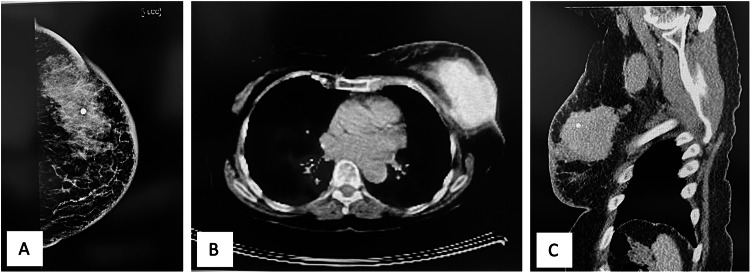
Left breast locally advanced TNBC. A: Left breast mammogram. B: PET-CT showing extensive FDG uptake in the left breast. C: Sagittal view CT scan showing left breast lesion. FDG: fluorodeoxyglucose; TNBC: triple-negative breast cancer.
